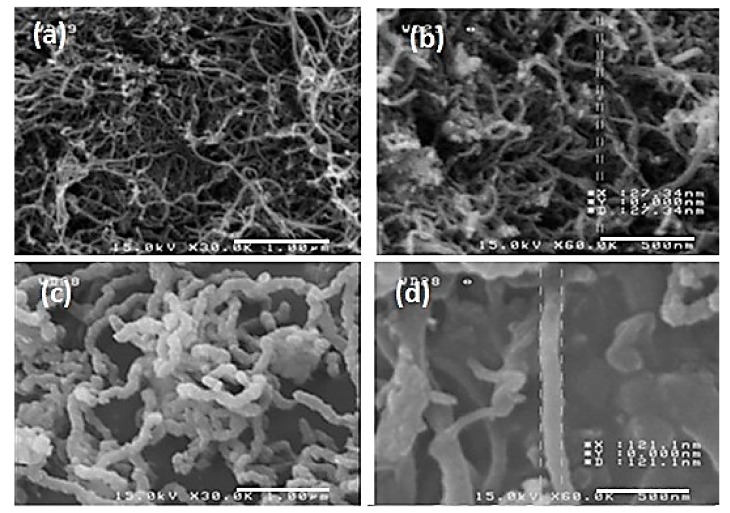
SEM image of pristine CNTs (a,b) using ultrasonic bath (the scale bars represent 1 µm and 500 nm, respectively) and (c,d) using stirring heater (the scale bars represent 1 µm and 500 nm, respectively) (catalyst concentration = 4 wt %, hydrogen flow = 200 cm^3^/min, and temperature = 1000 °C).
